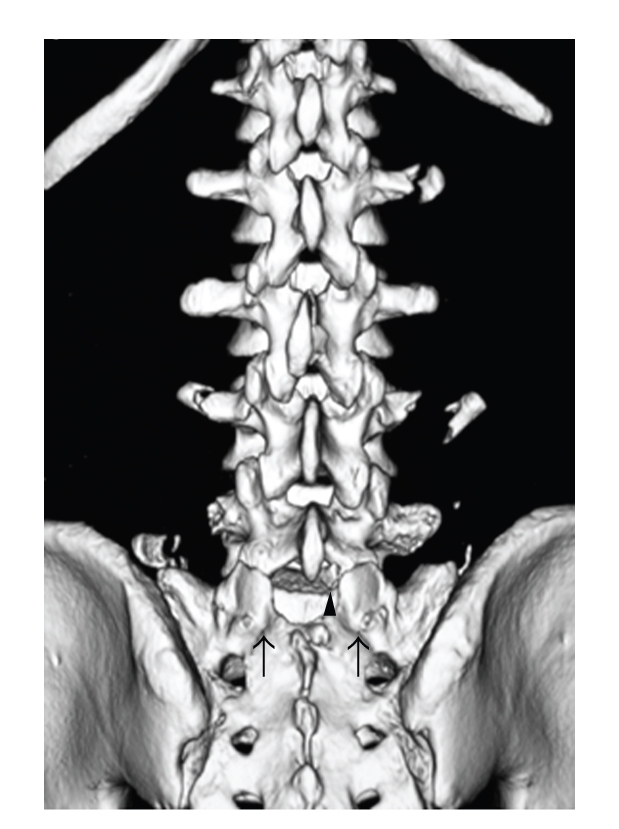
Reconstructed three-dimensional computed tomography demonstrating bilateral anterior facet dislocation with clearly visible sacral articular surfaces (arrows), the fractured tip of the right L5 inferior articular process (arrowhead), and transverse process fractures.
